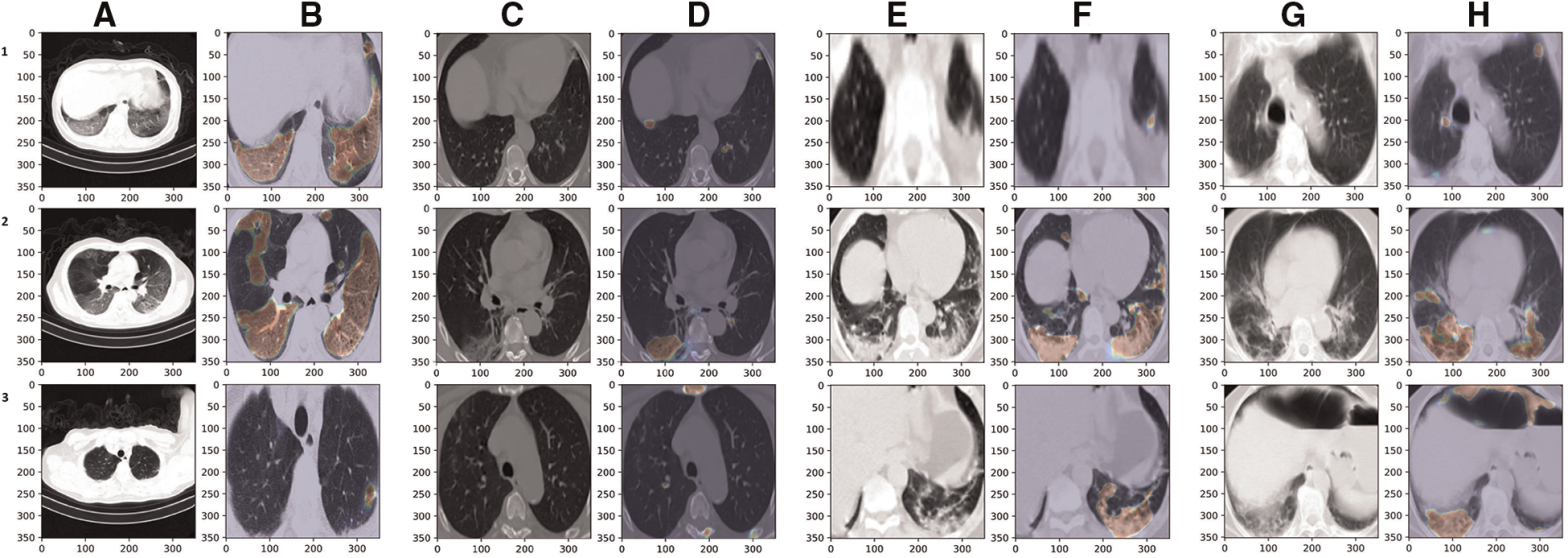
Axial CT study of four *randomly* sampled patients with COVID-19. Columns (A), (C), (E)/ (G) show the original images (columns C, E, / G segmented). Columns (B), (D), (F) / (H) show the bounding-box and lesion segmentation of the image at its left. Rows (1), (2) / (3) are different slices of the same patient at the start, middle and end of the study, respectively. The lesion quantification model determines the approximate lesion proportion, from top to bottom, as 59.11%, 56.49% / 11.30% for the figures on column (B); as 7.36%, 13.29% / 7.55% for those on column (D); as 15.61%, 40.72% / 38.11% for those on column (F); and as 11.73%, 27.91% / 79.97% for the slices on column (H). Note the patient on column (C), where the top and bottom slices show very little or no sign of lesions. That is the reason why it is necessary to process the complete CT volume. The Supplementary Material contains the fully processed studies for the four patients presented above.
